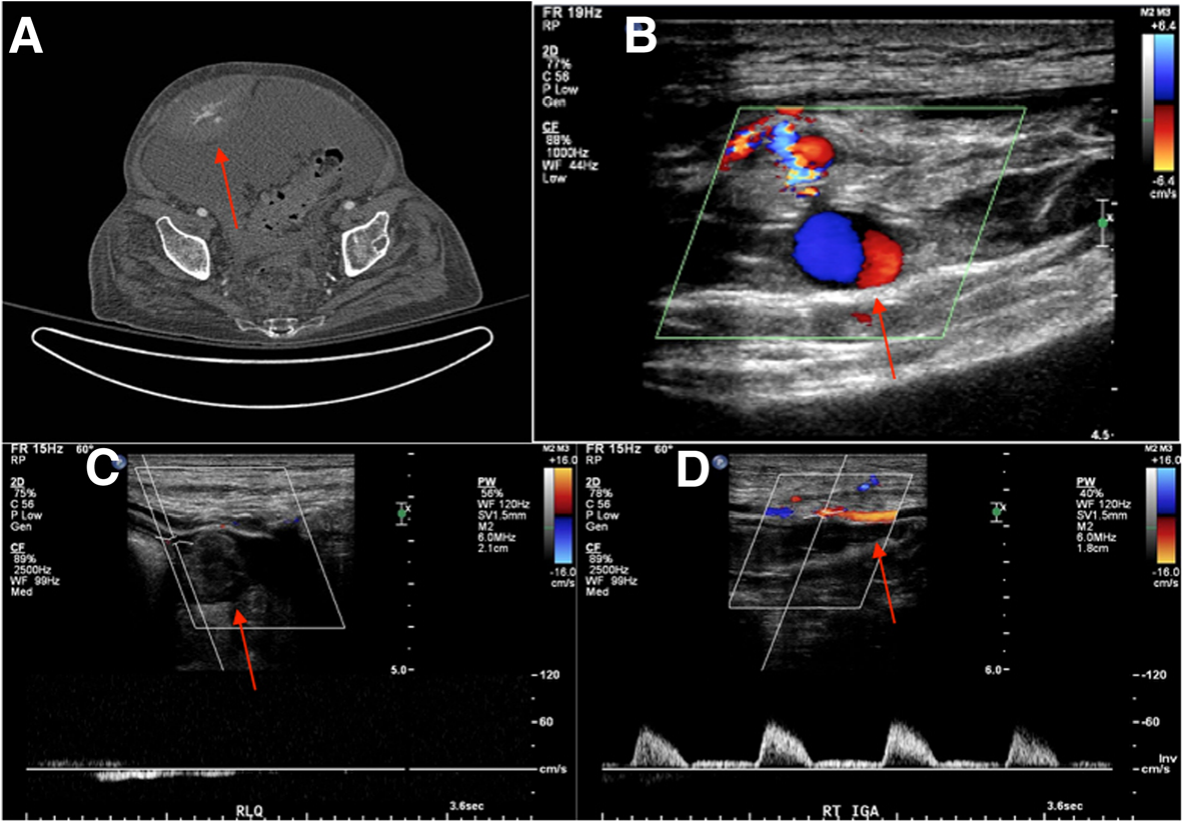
a CT angiography of the abdomen and pelvis demonstrates an area of active contrast extravasation within a large right rectus sheath hematoma. b Color Doppler ultrasound of the area of active contrast extravasation on the prior CT angiogram demonstrates turbulent color flow in a pattern consistent with a pseudoaneurysm. c Follow up ultrasound after thrombin injection into the pseudoaneurysm demonstrates persistent occlusion of the pseudoaneurysm. d There is preserved patency of the right inferior epigastric artery
